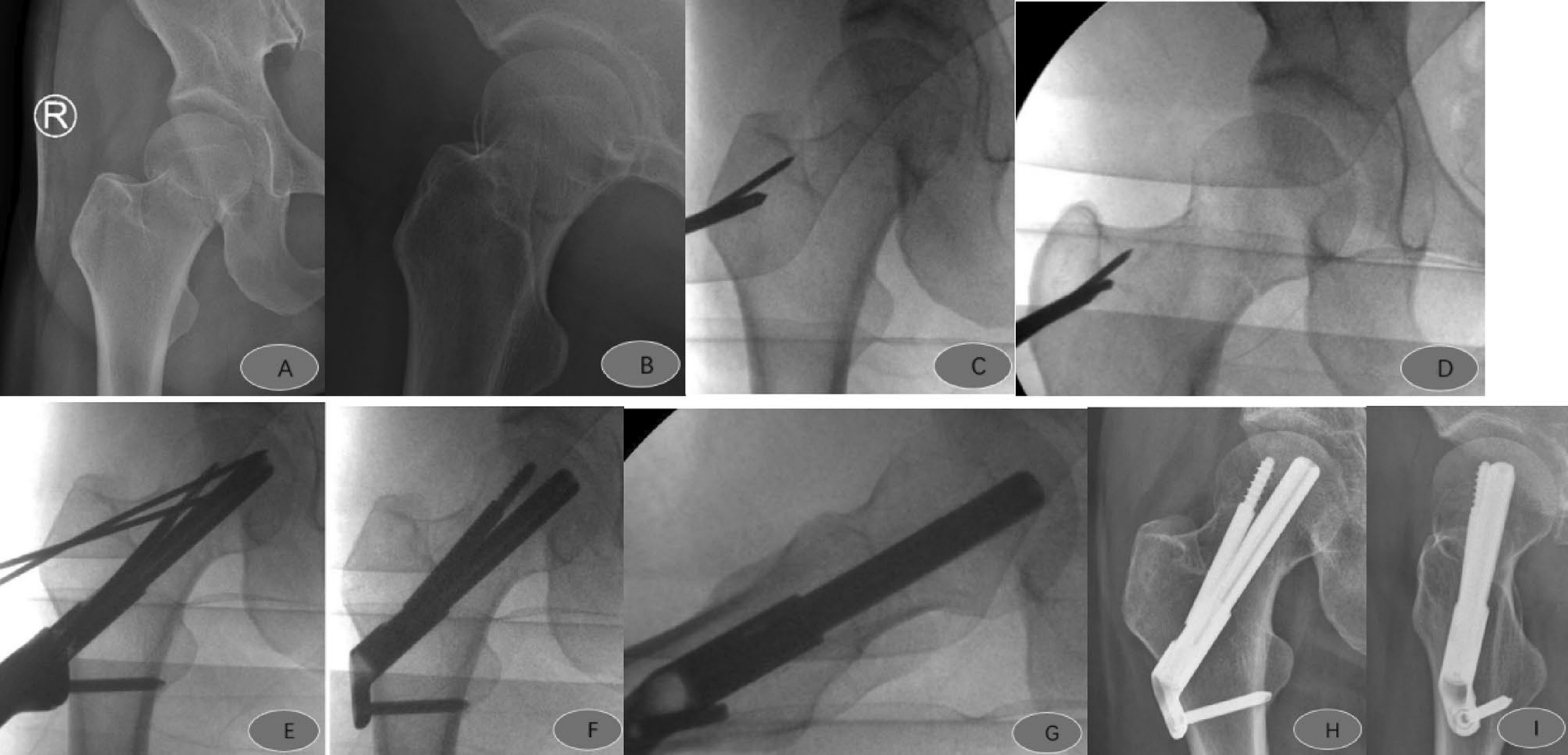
Female, 43 years old, admitted to the hospital with right hip pain and limited movement due to a fall; preoperative anteroposterior (A) and lateral (B) views show a right femoral neck fracture, Garden type I, type 2a; FNS was placed after satisfactory repositioning 5 days after the injury; intraoperative operations are shown in Figures (C, D, E, F and G). Postoperative anteroposterior (H) and lateral (I) radiographs 3 months after surgery show good fracture healing and satisfactory internal fixation position.
